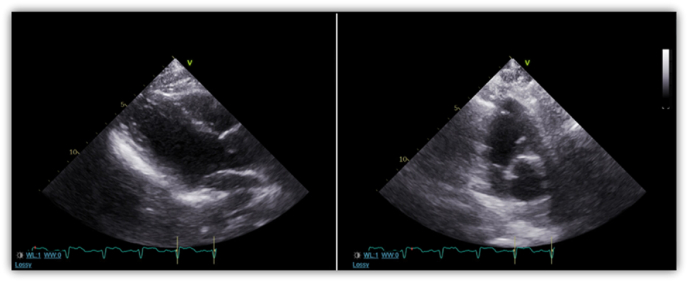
Transthoracic echocardiogram (TTE) demonstrated basal ventricular ballooning, indicating rTTC.
